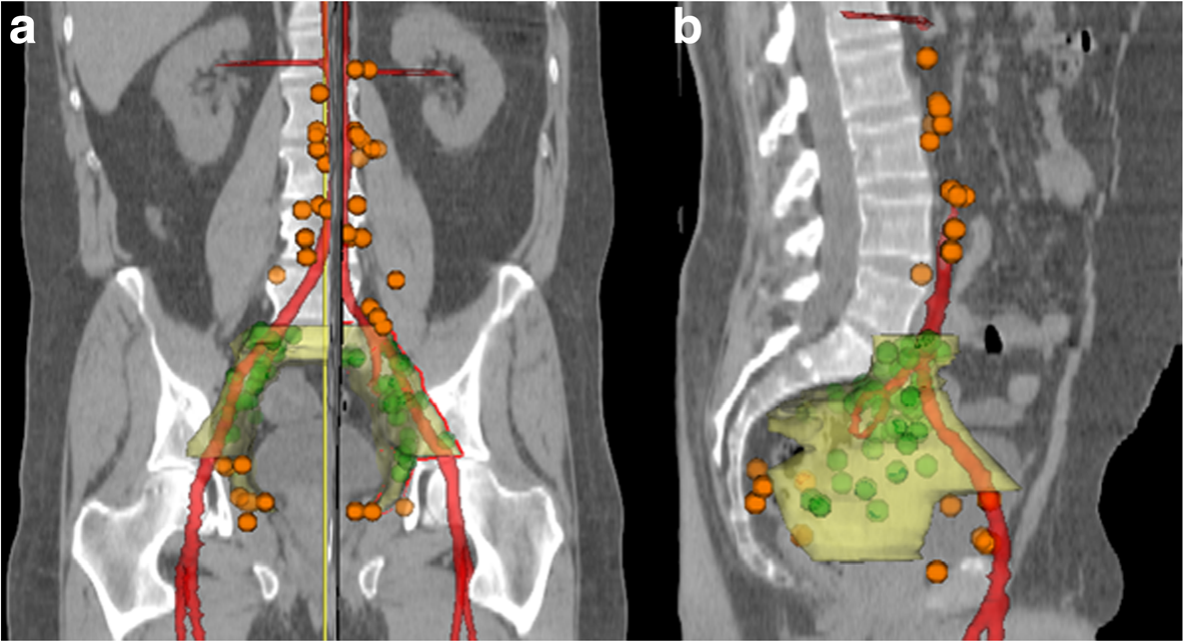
Image: Frontal (a) and lateral (b) overview depicting color-coded lymph nodes detected by PSMA-PET imaging that would have been inside (green) or outside (orange) of a standard clinical target volume (yellow) by RTOG consensus
